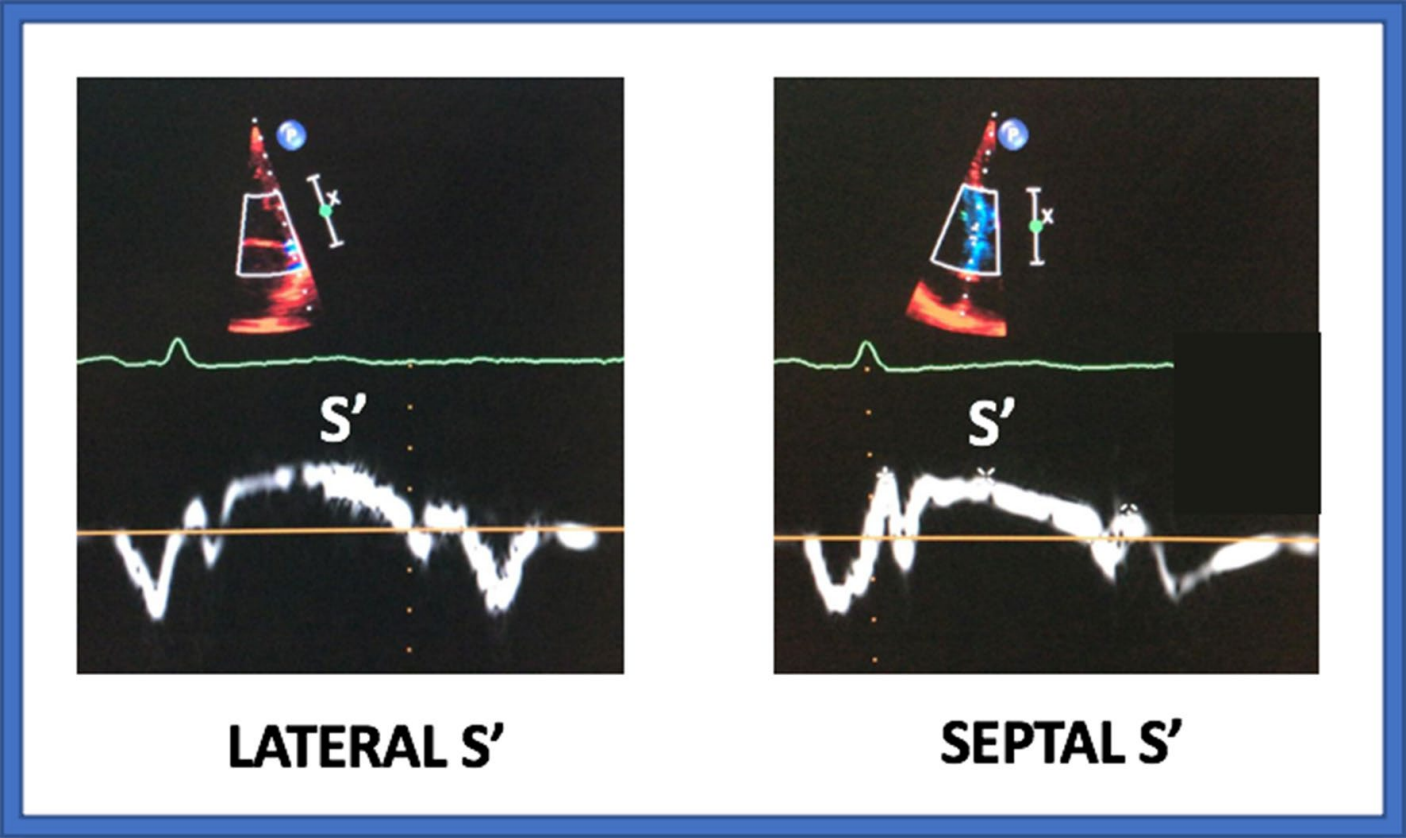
Measurement of peak systolic (S’) velocities by 2-dimensional tissue Doppler imaging. S’ represents the peak myocardial longitudinal systolic shortening velocity during ejection assessed by TDI. S′ velocities are measured at the level of the lateral (left panel) and medial (right panel) mitral annulus by pulsed-wave TDI from the apical four-chamber view. Average S’ is calculated as the mean of the lateral and medial S’ velocities. TDI is performed adjusting gain and frame rate to get an appropriate tissue characterization
